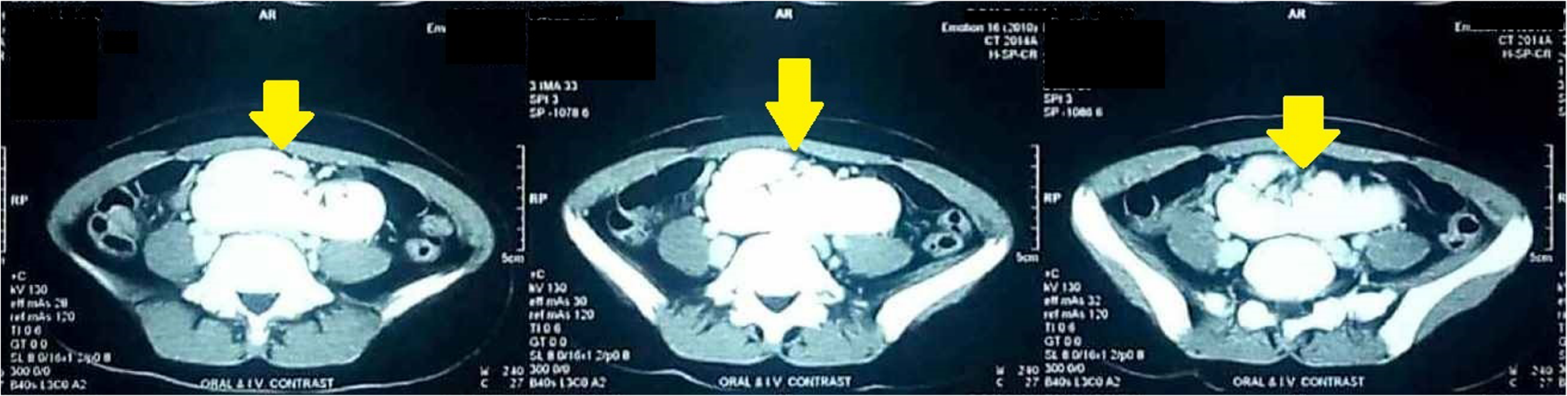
Multi-detector computed tomography scan showing the centrally placed pancake kidney (*yellow arrows* in transverse section)
